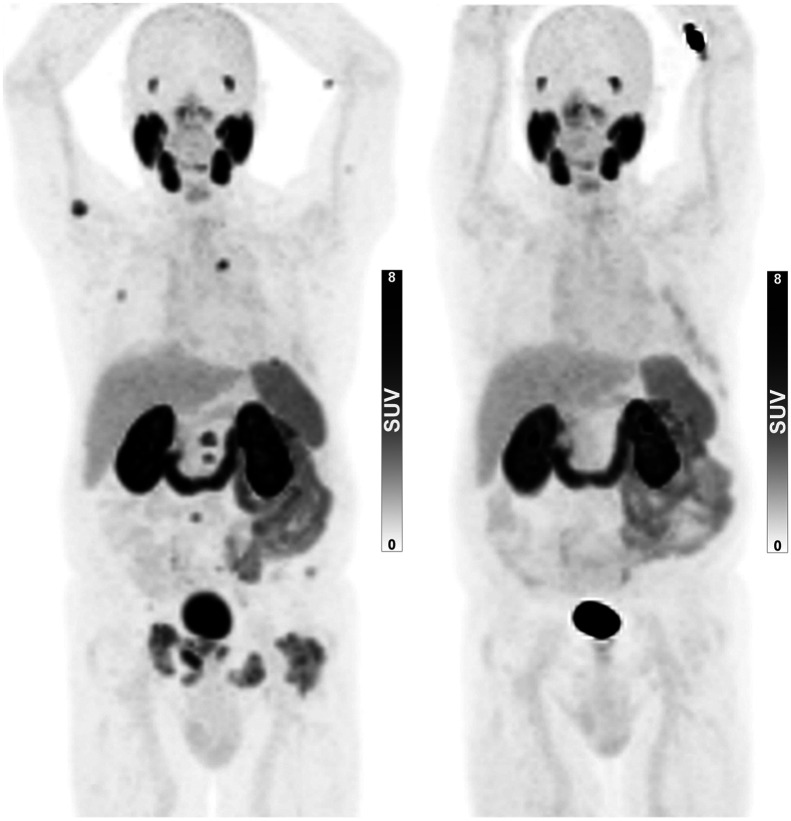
^68^Ga-PSMA-11 PET/CT scans of 83-y-old B(−) man before (left) and after (right) initiation of hormonal therapy. Staging scan (left) was performed without preimaging biopsy, revealing advanced disease. On imaging performed 10 mo later (right), all previous PSMA-positive lesions demonstrated no uptake of ^68^Ga-PSMA-11. PSA level of this patient decreased dramatically on therapy initiation as well (from 67 to 3.1 ng/mL).
